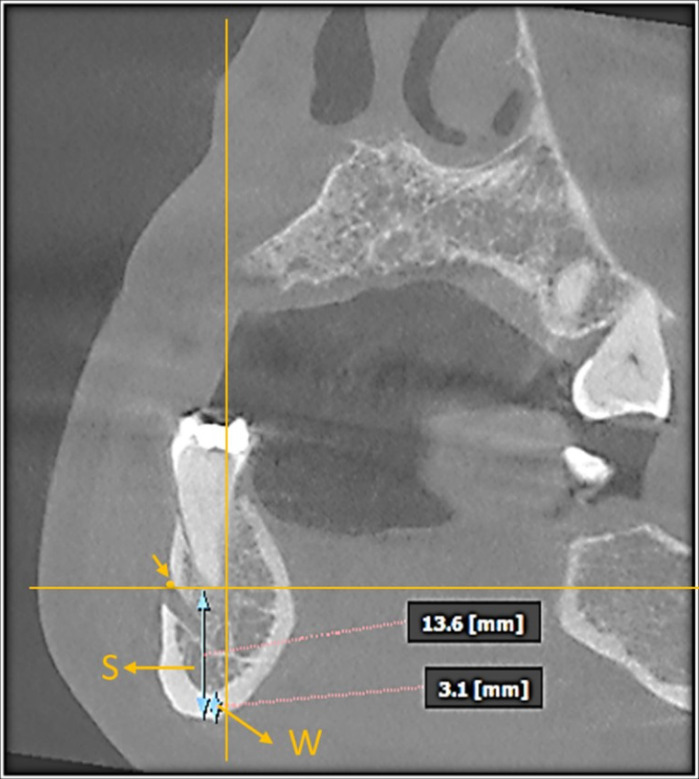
CTI(S) - computed tomography mandibular index (superior).
